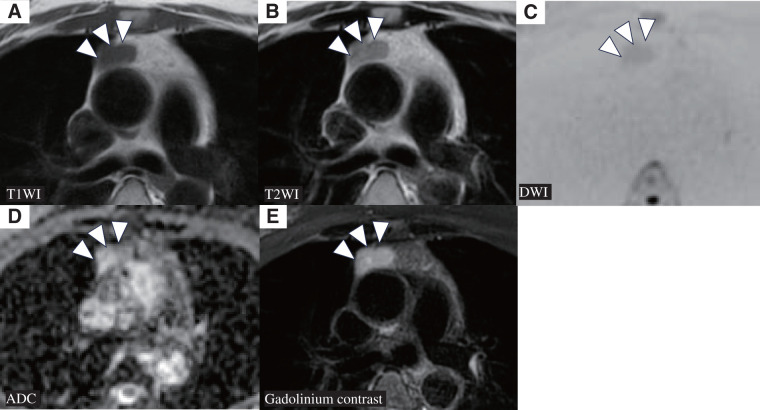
Contrast-enhanced chest MRI findings. (A) T1-weighted imaging showing uniform low signal intensity. (B) T2WI demonstrating pale, homogeneous high signal intensity without fat suppression. (C, D) DWI presenting pale high signal intensity without ADC reduction. (E) Gadolinium-enhanced MRI exhibiting mild enhancement limited to the capsule-like peripheral structure, with no internal enhancing component. ADC: apparent diffusion coefficient; DWI: diffusion-weighted imaging; MRI: magnetic resonance imaging; T2WI: T2-weighted imaging
